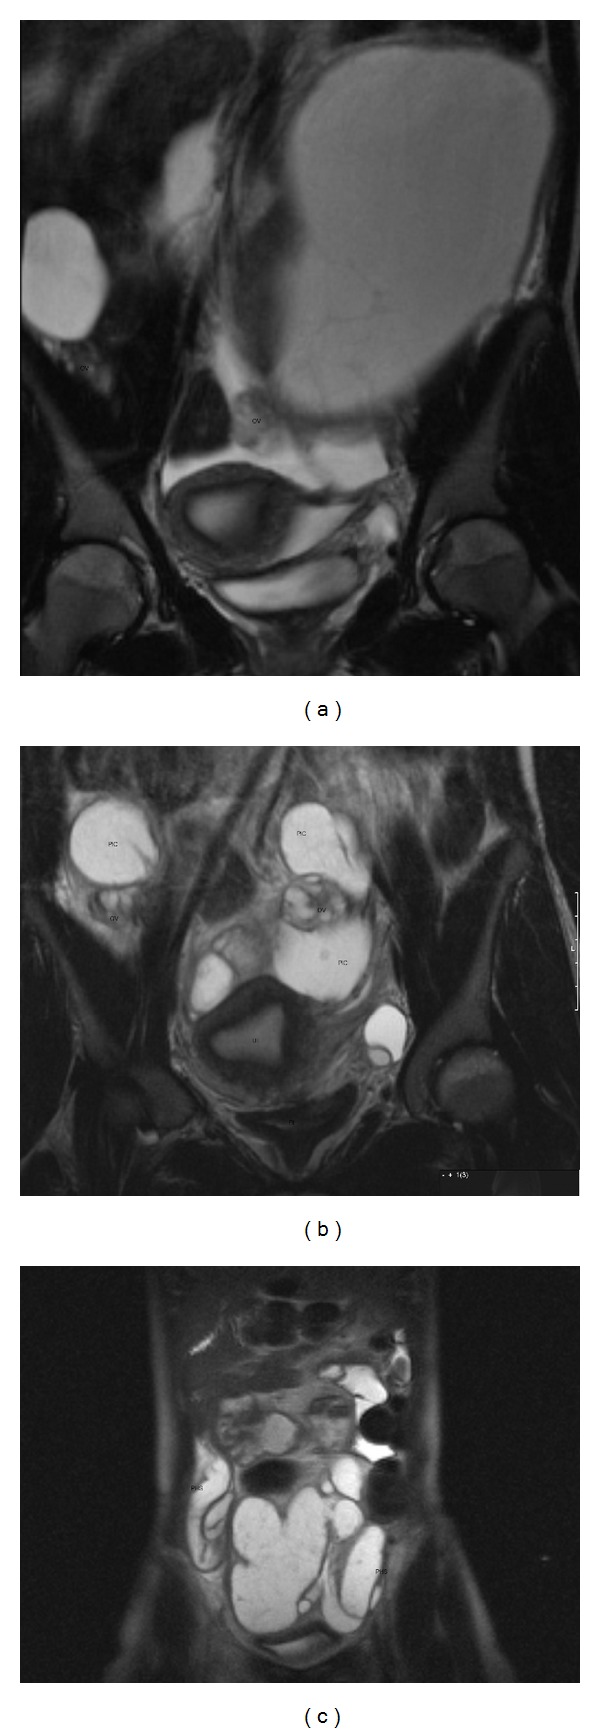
(a) Coronal T2 MR image demonstrating both ovaries (Ov) surrounded by cystic fluid collections. (b) Coronal T2 MR image shows both ovaries (Ov) surrounded by peritoneal inclusion cysts (PIC) after drainage. UT = uterus. Bl = bladder. (c) Coronal T2 MR image demonstrating a “pseudohydrosalpinx” (PHS) bilaterally. “Spider web” septations are seen within the peritoneal inclusion cyst that has decreased in size after drainage.
